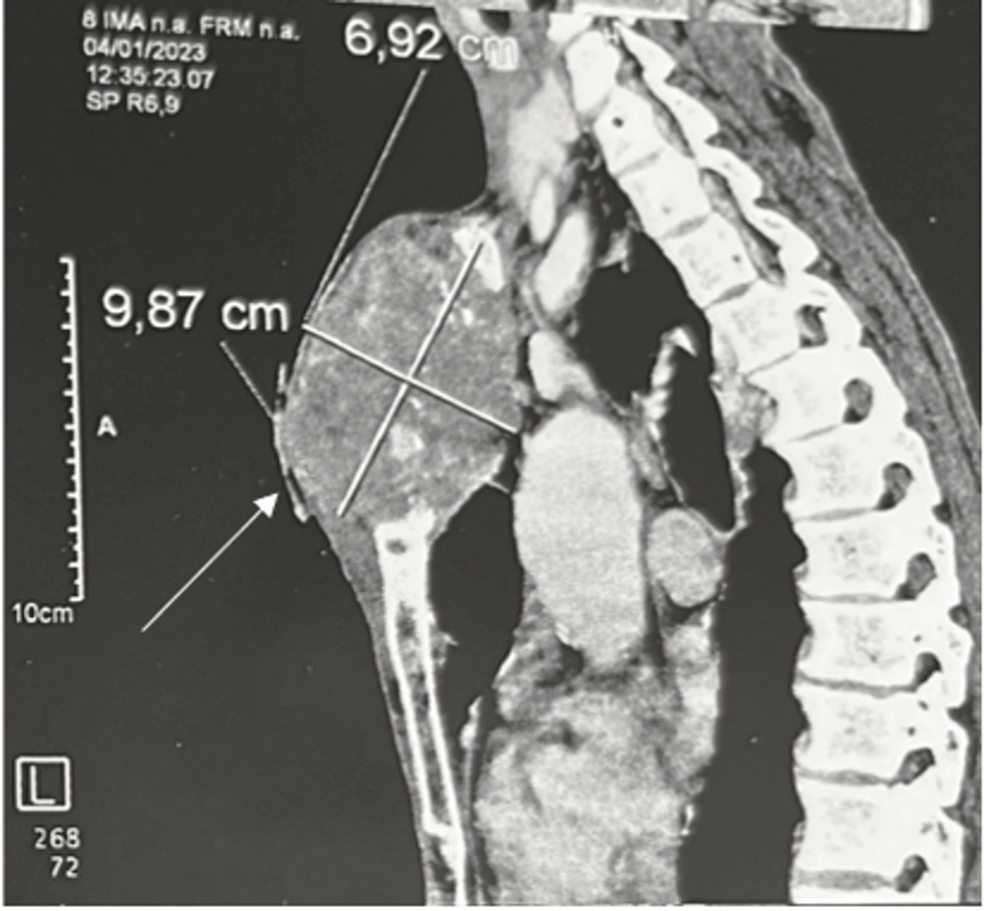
Chest CT scan showing a sternal osteolytic lesion with a significant extension to the adjacent soft tissues (6x9 cm; white arrow)
